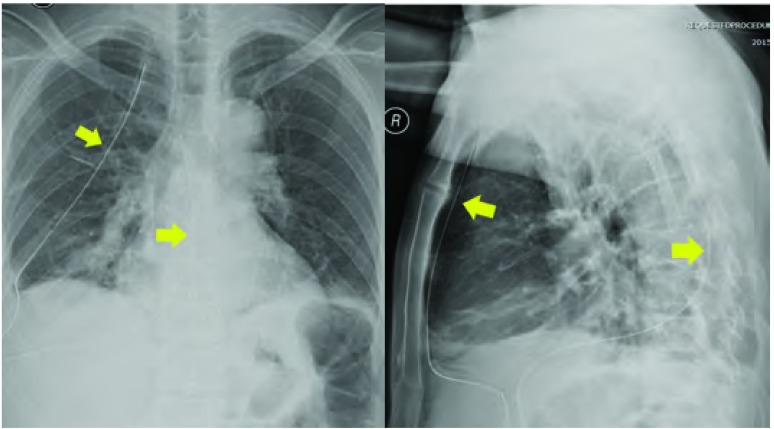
单孔VATS术后留置16 F胸腔闭式引流管，引流效果良好。A：术后胸部正位X线结果；B：术后胸部侧位X线结果。 Chest drainage with two 16 F chest tubes after Uni-VATS got a unobstructed drainage. A: X-ray findings post-operation on thoracic positive position; B: X-ray findings post-operation on thoracic lateral position.
